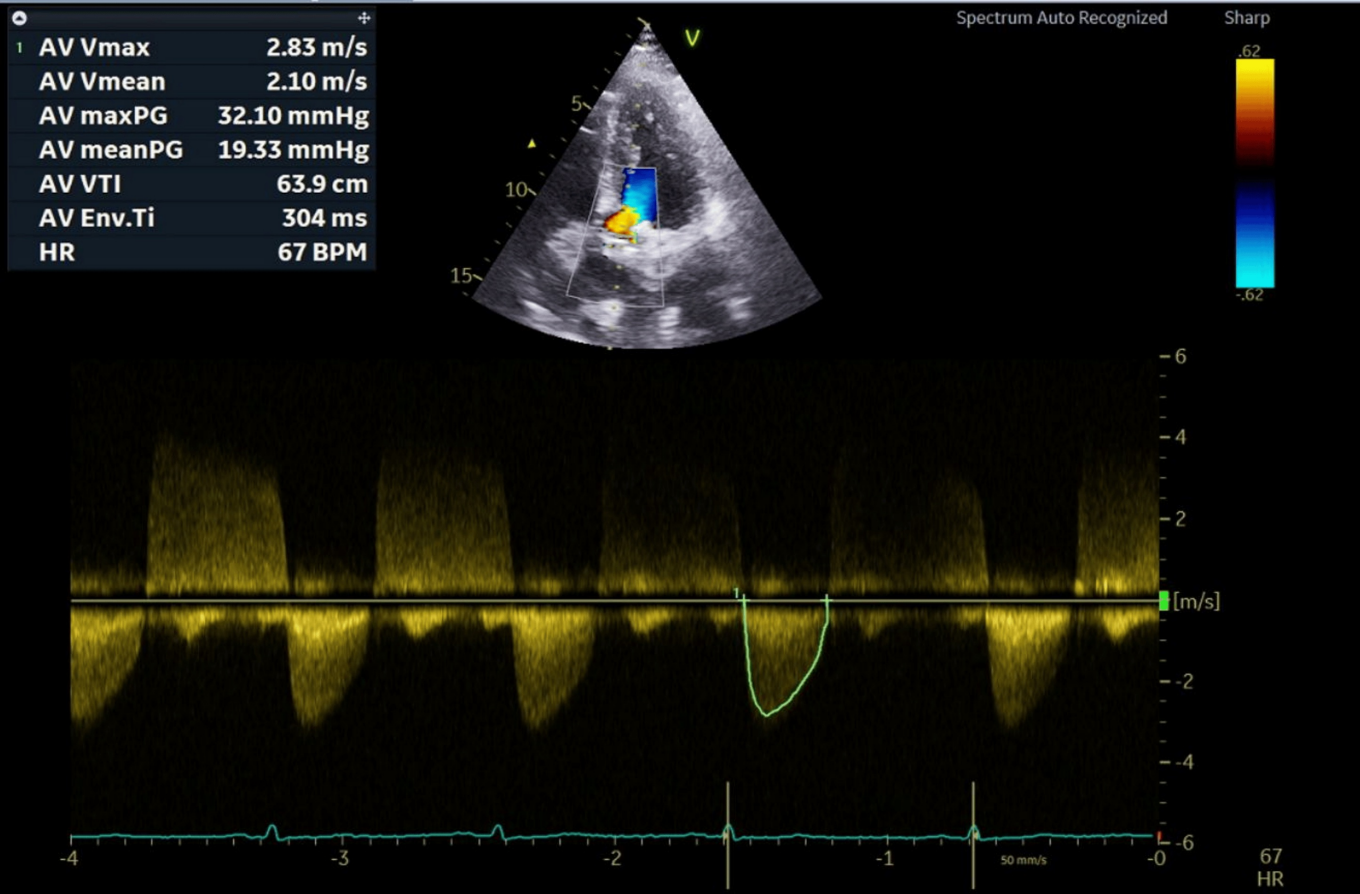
Echocardiogram, apical five-chamber view, showing mild aortic stenosis and mild aortic regurgitation
